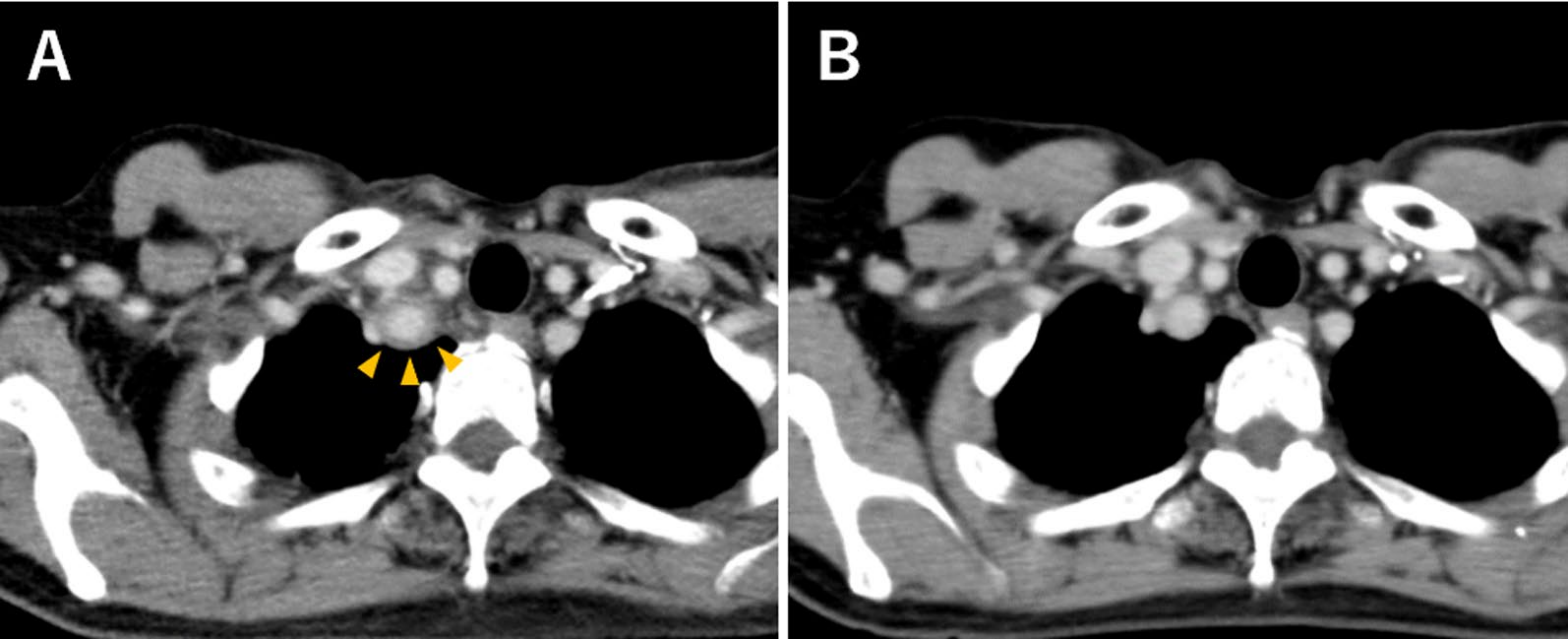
CT scan findings: the right subclavian artery. A Wall thickening and peri-aortic soft tissue infiltration of the right subclavian artery on day 11 after pegfilgrastim administration (orange arrowheads). These findings were improved on day 26 (B)
